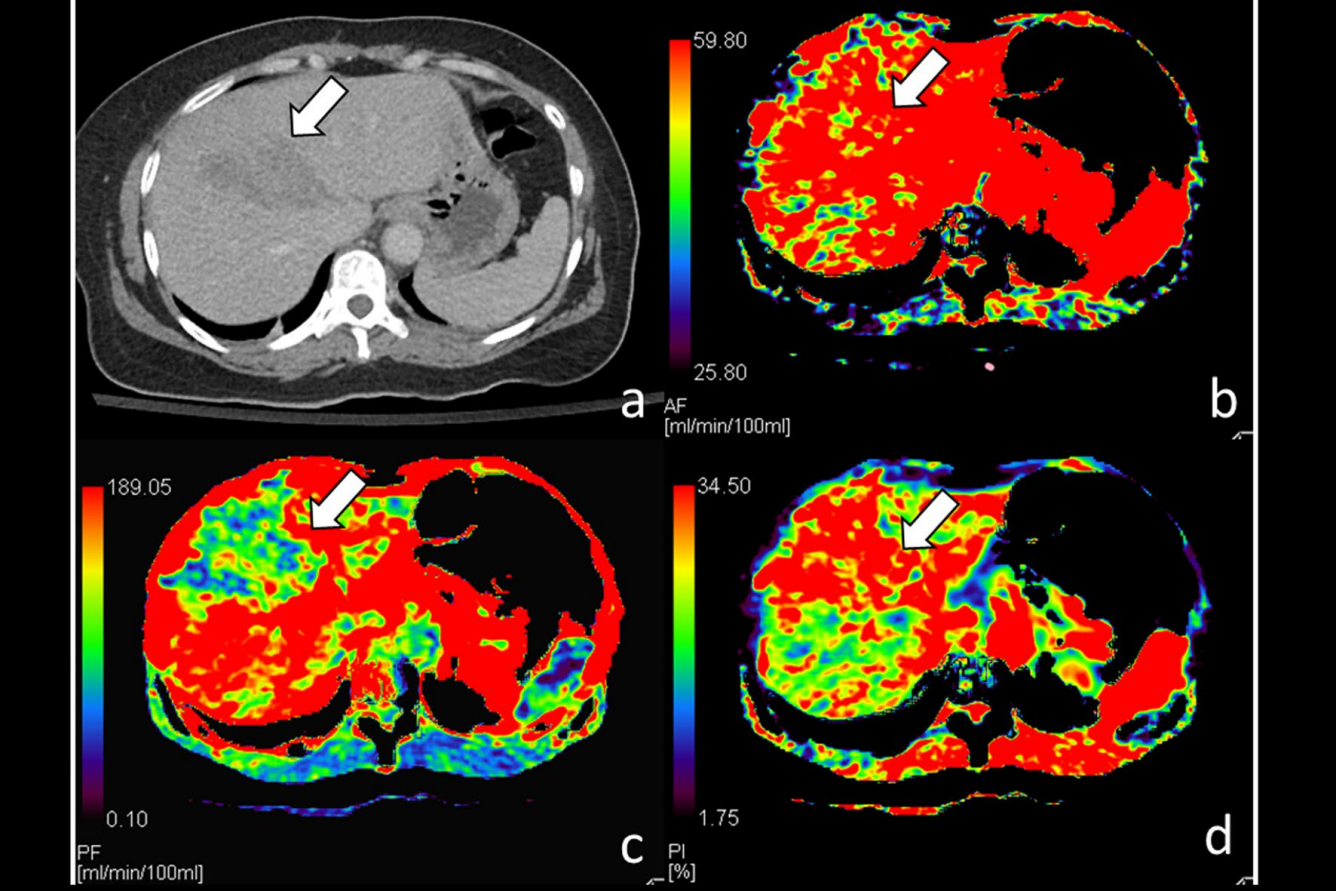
CT of a 56-year-old woman with grade III liver injury at segment IV receiving observation management only. (a) Contrast-enhanced CT of the liver shows focal injury at segment IV (arrow); (b) hepatic arterial perfusion CT does not show arterial flow defect (arrow) but (c) hepatic portal venous perfusion CT reveals decreased portal venous flow at traumatic parenchyma (arrow); (d) CT perfusion shows compensatory increase of hepatic perfusion index at segment IV (arrow).
